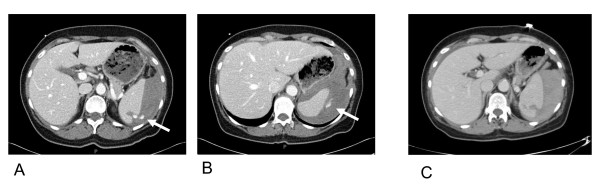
CT scans from the outside hospital demonstrate contrast extravasation from the spleen (A,B). Repeat imaging at Denver Health reveals the blush has resolved (c).
